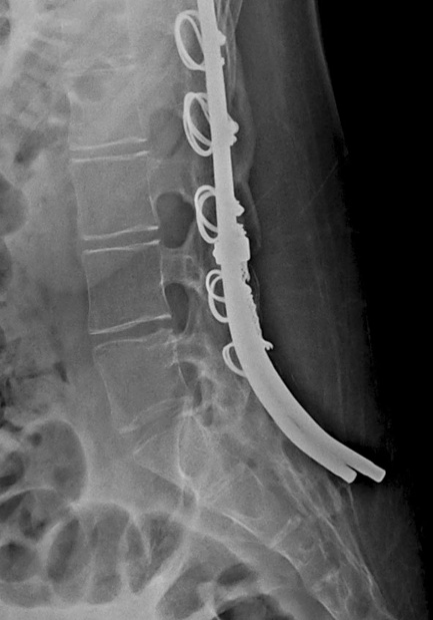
Lateral radiograph in patient with DMD shows rods that were too long and not firmly positioned against the posterior surface of the sacrum. Relief occurred after shortening of the rod ends. Note the solid continuous posterior bone fusion from the lumbar vertebrae across the lumbosacral joint onto the sacrum
